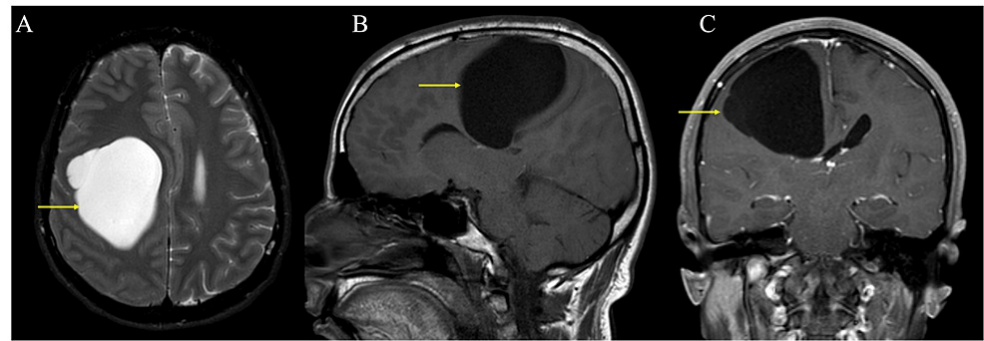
The axial T2 (A), sagittal T1 (B), and coronal CET1 (C) of brain MRI show an intra-axial clear fluid signal intensity cyst within the right posterior frontal lobe (yellow arrows). There is a mass effect of the brain parenchyma and communication with the lateral ventricle. No solid components or abnormal enhancement was noted within the cyst. All the features indicate a diagnosis of a neuroepithelial cyst.
